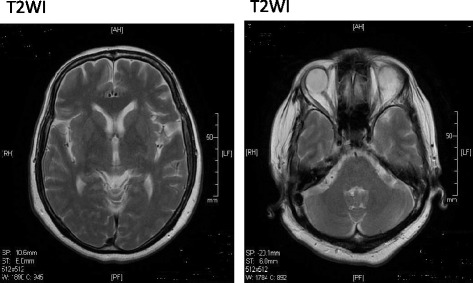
Brain magnetic resonance images of the patient at 57 years of age (2013). T2-weighted images (T2WI) demonstrated no remarkable abnormalities, including fresh cerebral infarction, bleeding, space-occupying lesions, and prominent atrophic changes in brain regions including the bilateral putamen. Moreover, there was no high-intensity signal of the lateral rim of the putamen
